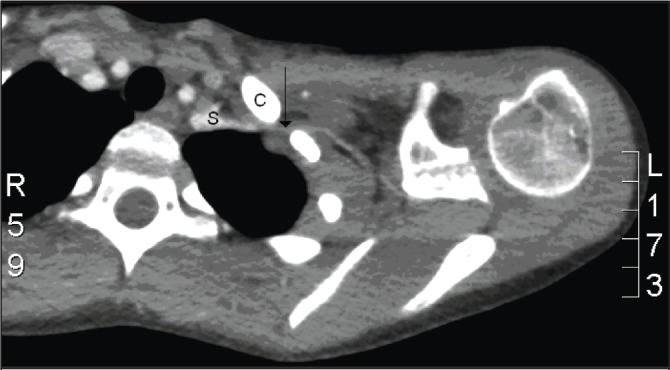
Computed tomography angiography showing the medial clavicle (C) compressing the subclavian artery (S) with significantly reduced volume (arrow)
